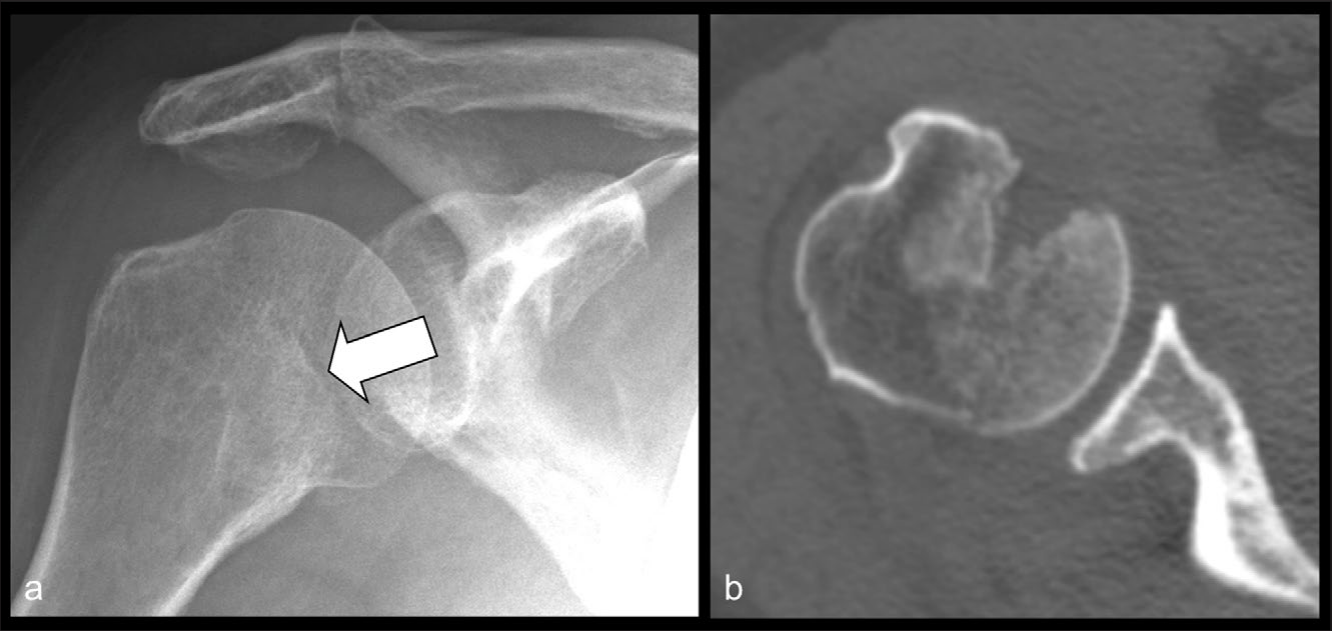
Patient with history of posterior shoulder dislocation. Radiograph (a) showing abnormal line on the humeral head (arrow). At CT, the deformity of the humeral head corresponds to the shape of the posterior margin of the glenoid.
